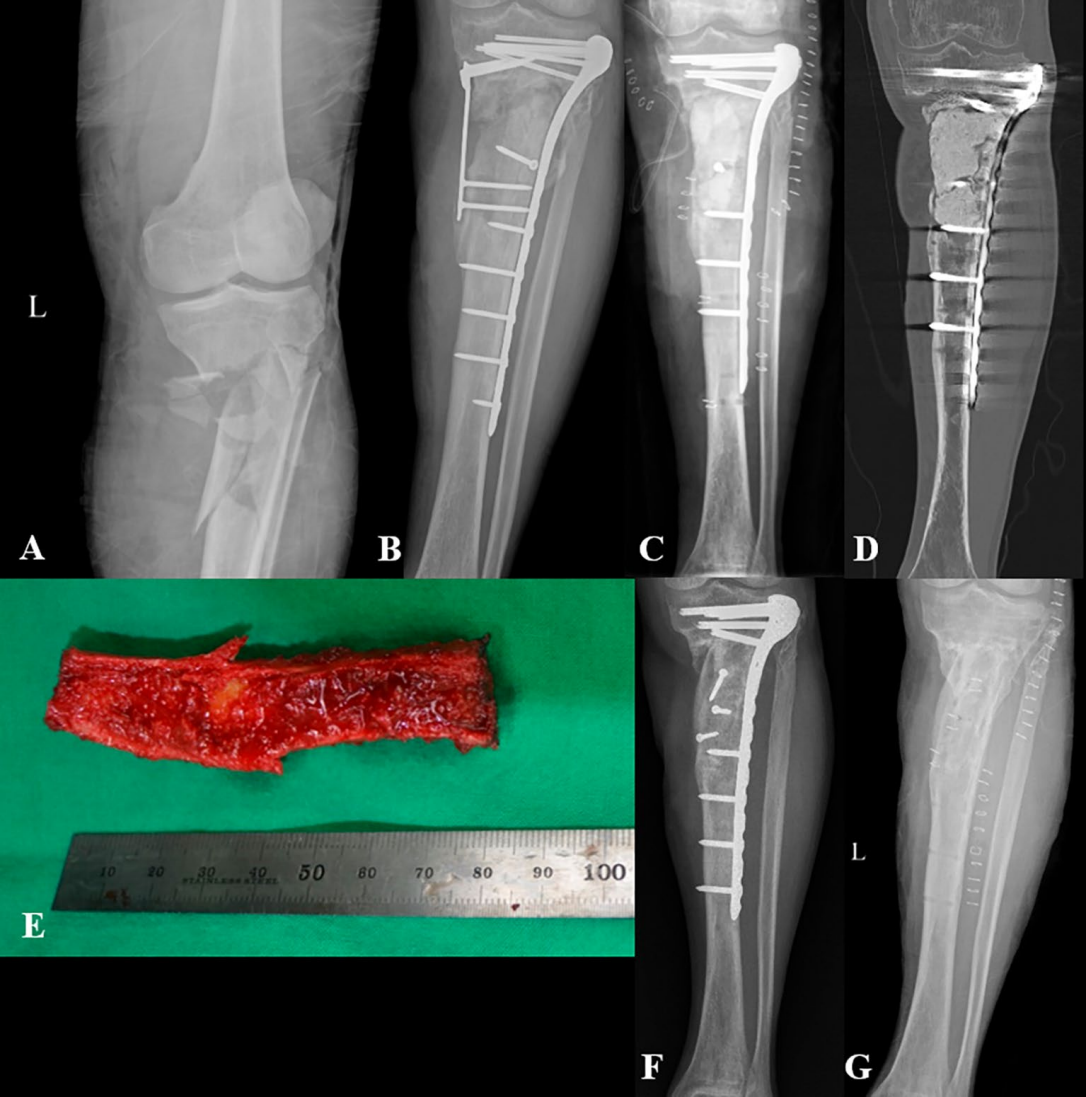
(A) 49-year-old male patient had a Gustilo-Anderson type III B open fracture of the left proximal tibia due to a motorcycle accident. (B) The fracture was treated with open reduction and internal fixation after debridement, and a rotation flap was performed for the skin defect. (C) At 9 months after surgery, surgical site infection was diagnosed, and antibiotic-loaded bone cement was inserted with plate fixation after serial debridement. (D) A coronal computed tomography image shows the cement spacer inserted into the bone defect. The bone defect length was measured to be 7.5 cm. (E), (F) Eight weeks after the insertion of the cement spacer, the infection resolved, and autogenous iliac bone grafting was performed. An iliac strut bone was harvested 1 cm longer than the bone defect. Note that there is sufficient cancellous portion contained within the strut bone. (G) The patient achieved a complete bone union without complications.
